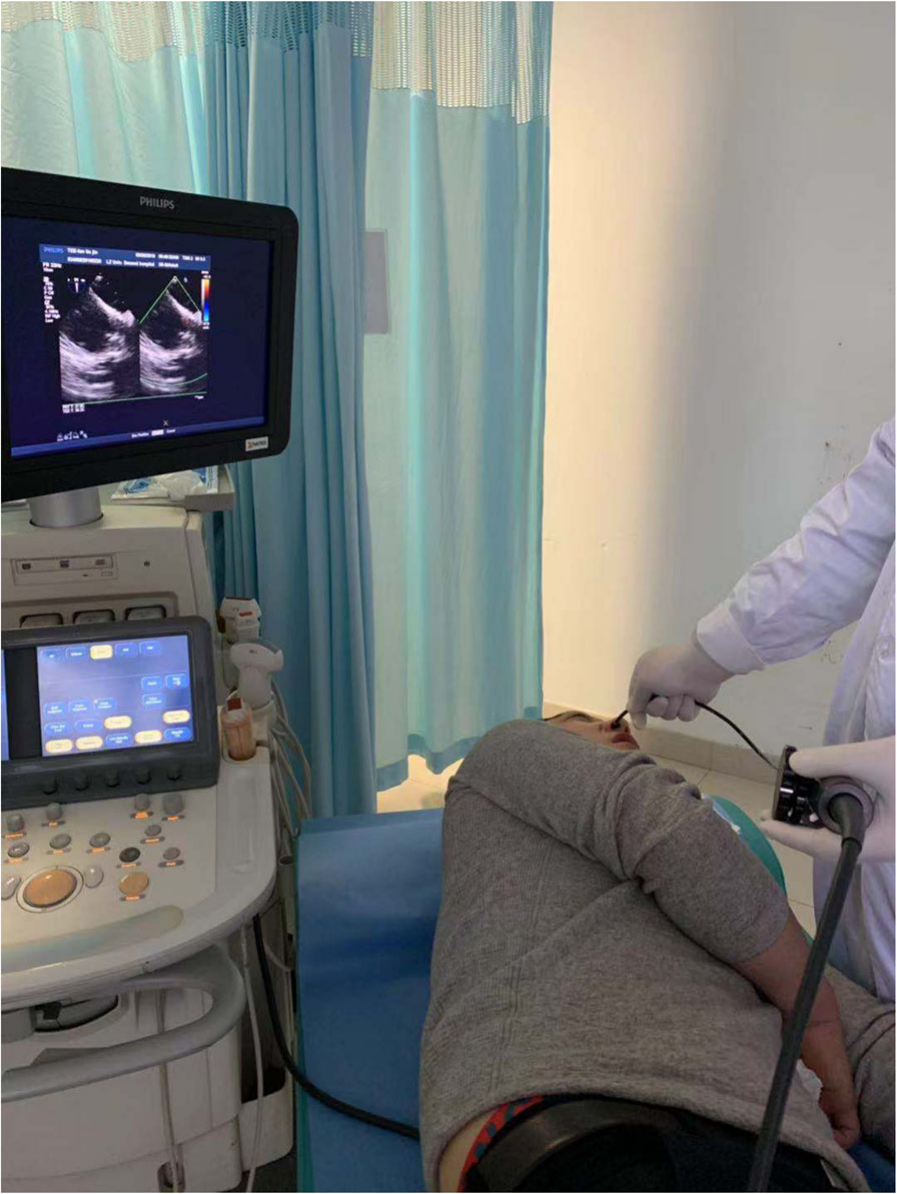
Transnasal echocardiography
